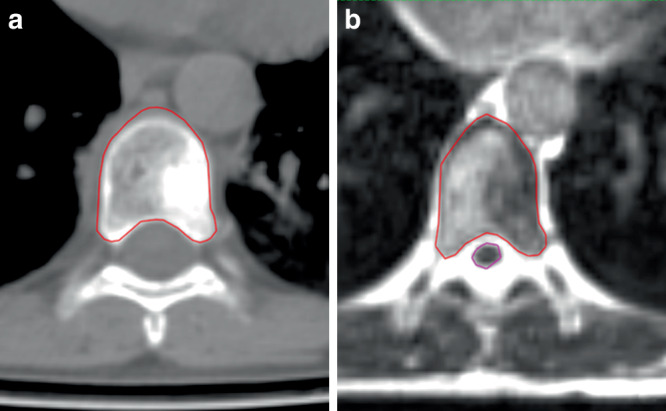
Delineation of CTVs and spinal cord on CT and MRI images. a Axial view of the CTV contour (red); b axial MRI slice of the CTV contour in red and spinal cord contour in violet. Note the spinal cord cannot be visualized on the CT image (a). *CTV* clinical target volume; *CT* computed tomography; *MRI* magnetic resonance imaging
